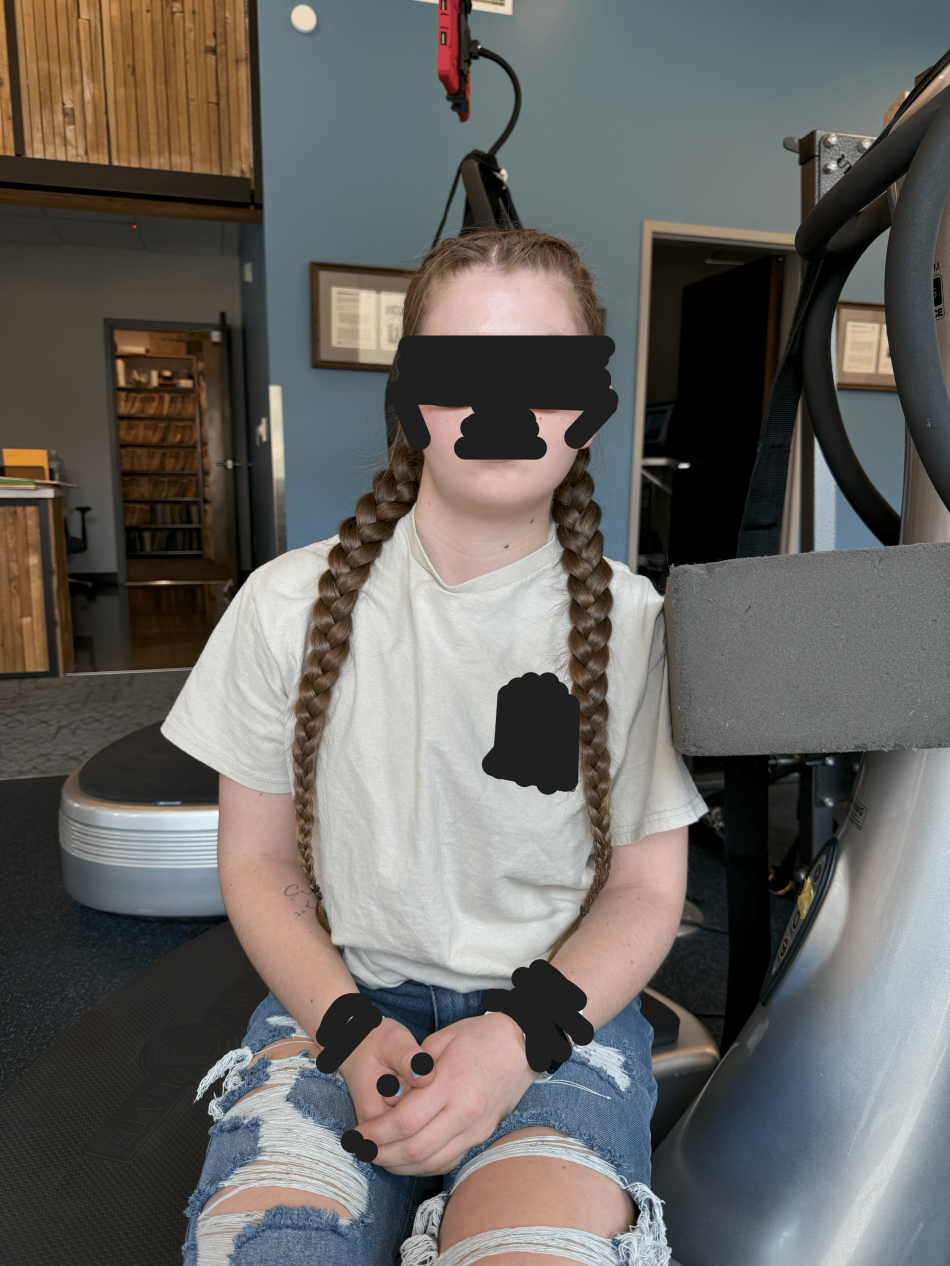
The patient is seated on the Power Plate® with a large block placed on the left shoulder. At eye level, she translates her head in the mirror image of her radiographs to strengthen the muscles and improve the posture toward the midline.
